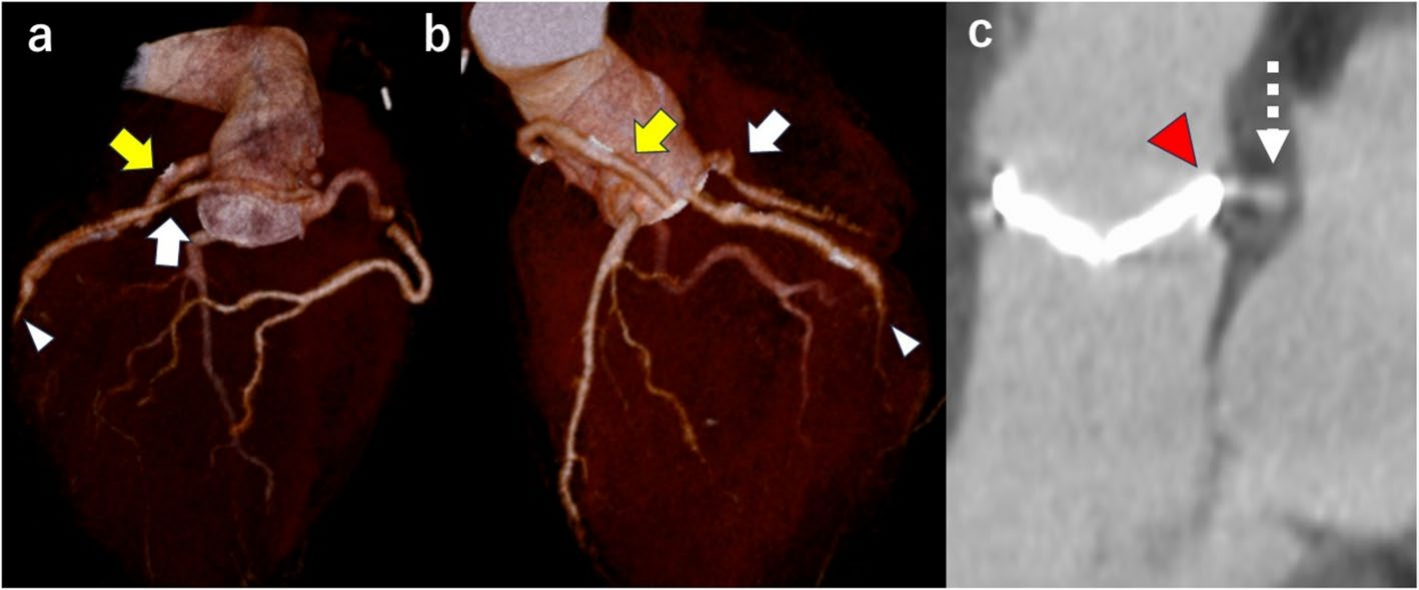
Postoperative computed tomography showing that the bypass graft (yellow arrow) to the obtuse marginal branch (white arrowhead) and anomalous left circumflex coronary artery (white arrow) were patent (a and b). The prosthetic valve (red arrowhead) and the anomalous left circumflex coronary artery (white dotted arrow) were positioned near each other at the posterior aspect of the aorta (c)
